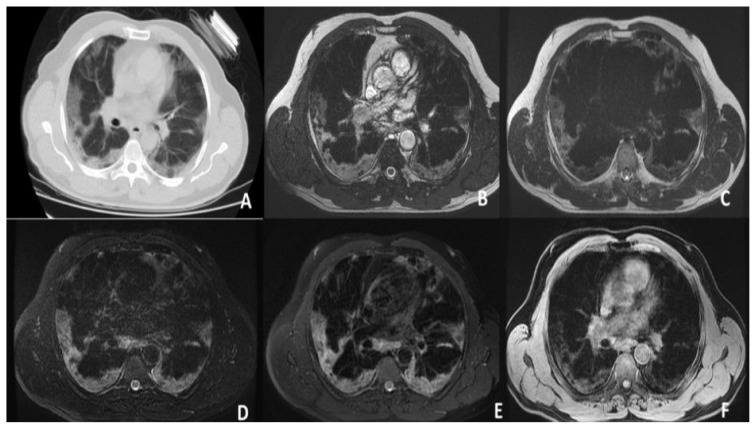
Bilaterally peripherally located GG and consolidation areas of the patient. A. CT. B. FIESTA sequence. C. SSFSE sequence. D. T2 FS sequence. E. T2 PROPELLER sequence. F. T1 LAVA-F sequence.
